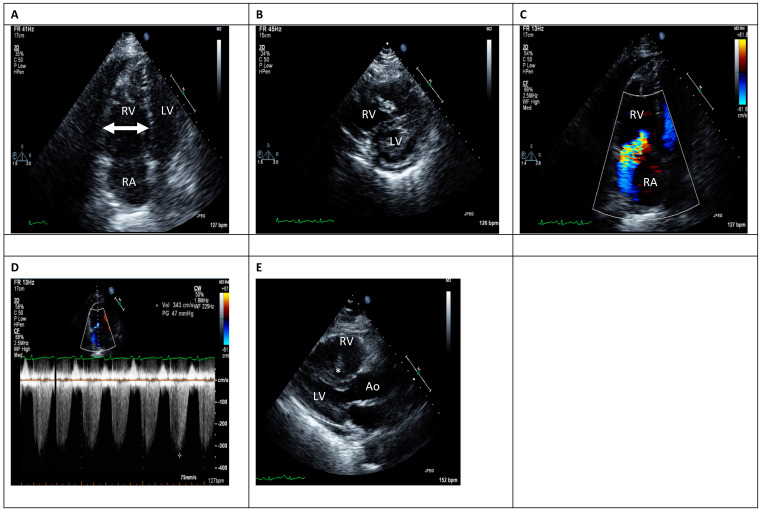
Echocardiographic findings suggestive of RV involvement in intermediate-risk PE. (A) Apical 4-chamber view showing right ventricular dilation. (B) Short-axis view showing interventricular septum deviation as well as a visible right-sided thrombus (asterisk). (C) Severe tricuspid regurgitation. (D) Pulmonary artery pressure Doppler curve demonstrating an increased pulmonary artery pressure. (E) Parasternal long-axis view showing a visible right-sided thrombus (asterisk). Ao—Aorta; LV—left ventricle; RA—Right atrium; RV—right ventricle.
